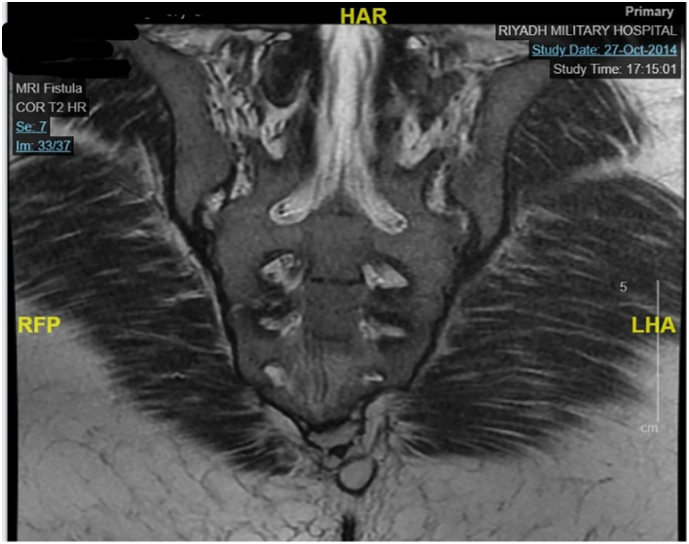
Pelvic magnetic resonance imaging (MRI) showing a pilonidal sinus.
